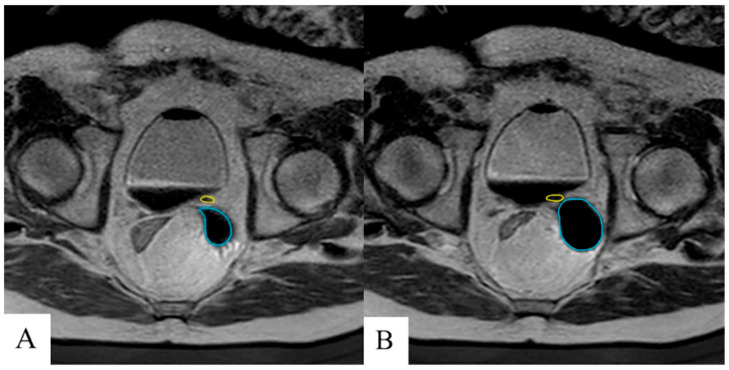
Near real-time T2 MRI to measure iceball growth (cyan) and proximity to the ureter (yellow) during early (A) and late (B) cryoablation in which a margin of 2 mm between the ureter and cryoablation border was maintained. Ureteral stent was left in place at discharge to maintain patency.
